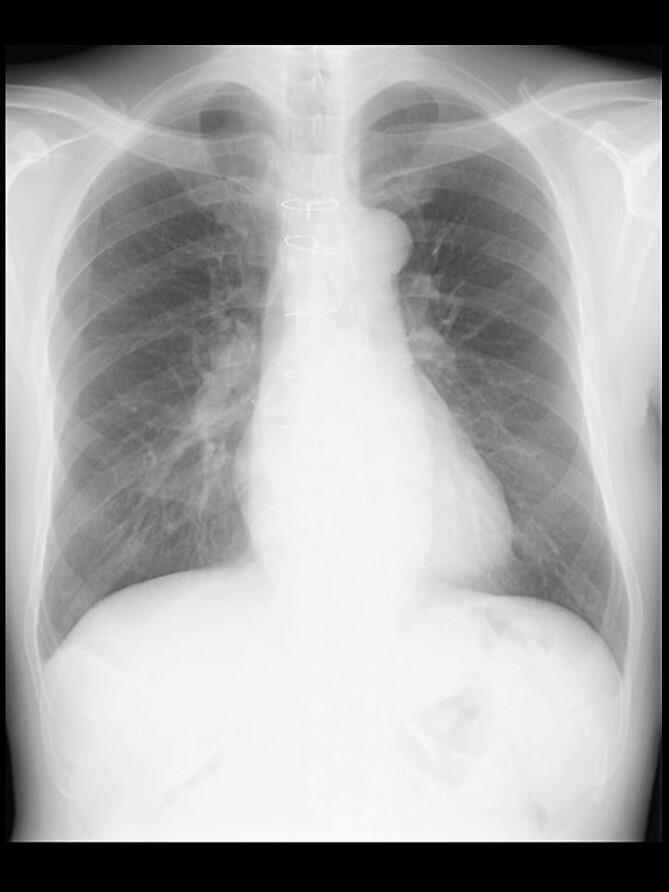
A chest radiograph in the upright position before surgery.
